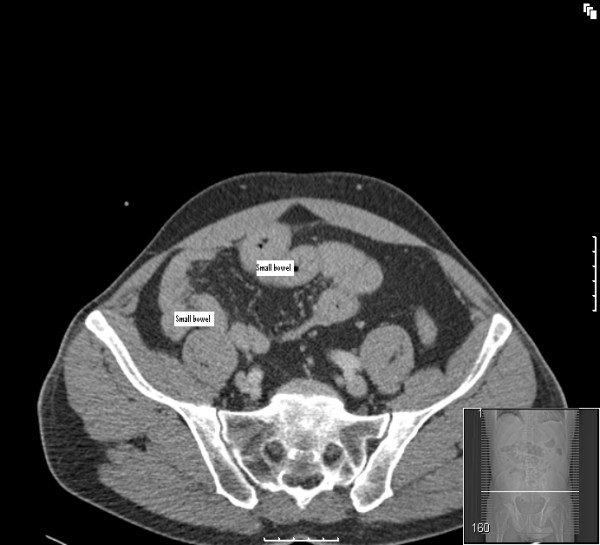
CT scan showing most of the small bowel on the right side of the abdomen.
